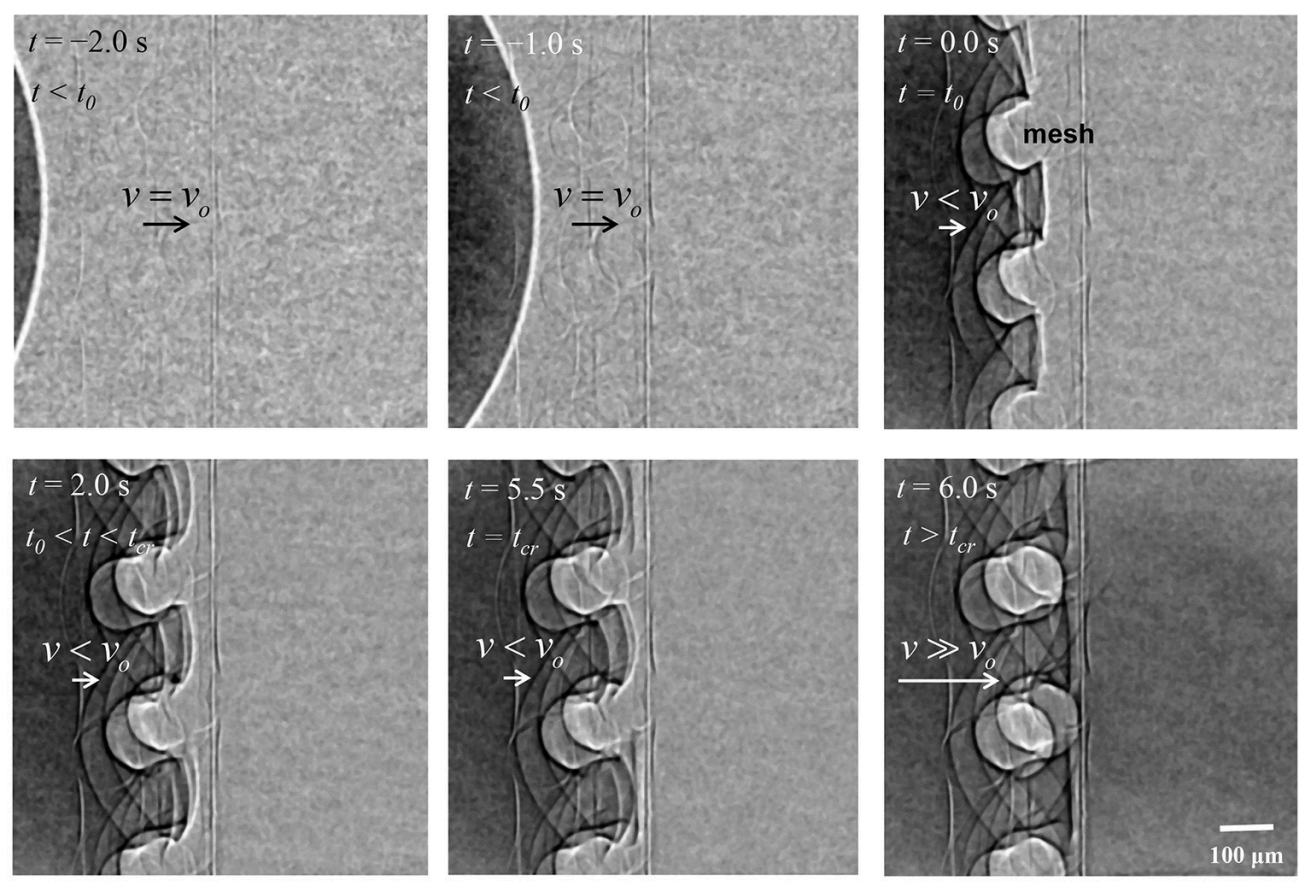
Typical X-ray images showing the water-filling flow in the xylem-inspired channel. Side view shows the movement of the water meniscus near the mesh structure. The water meniscus moved at a constant velocity *v*_0_ upon contact with the mesh structure at *t* = *t*_0._ The meniscus then split into smaller menisci between mesh pores. The menisci penetrated pores with a velocity lower than *v*_0_ and with increasing curvature until *t* = *t*_*cr*._ After the complete penetration of the water meniscus through the porous mesh, the menisci merged into a large meniscus, which then moved rapidly to the right side (*t* > *t*_*cr*)_. Scale bar = 100 μm.
